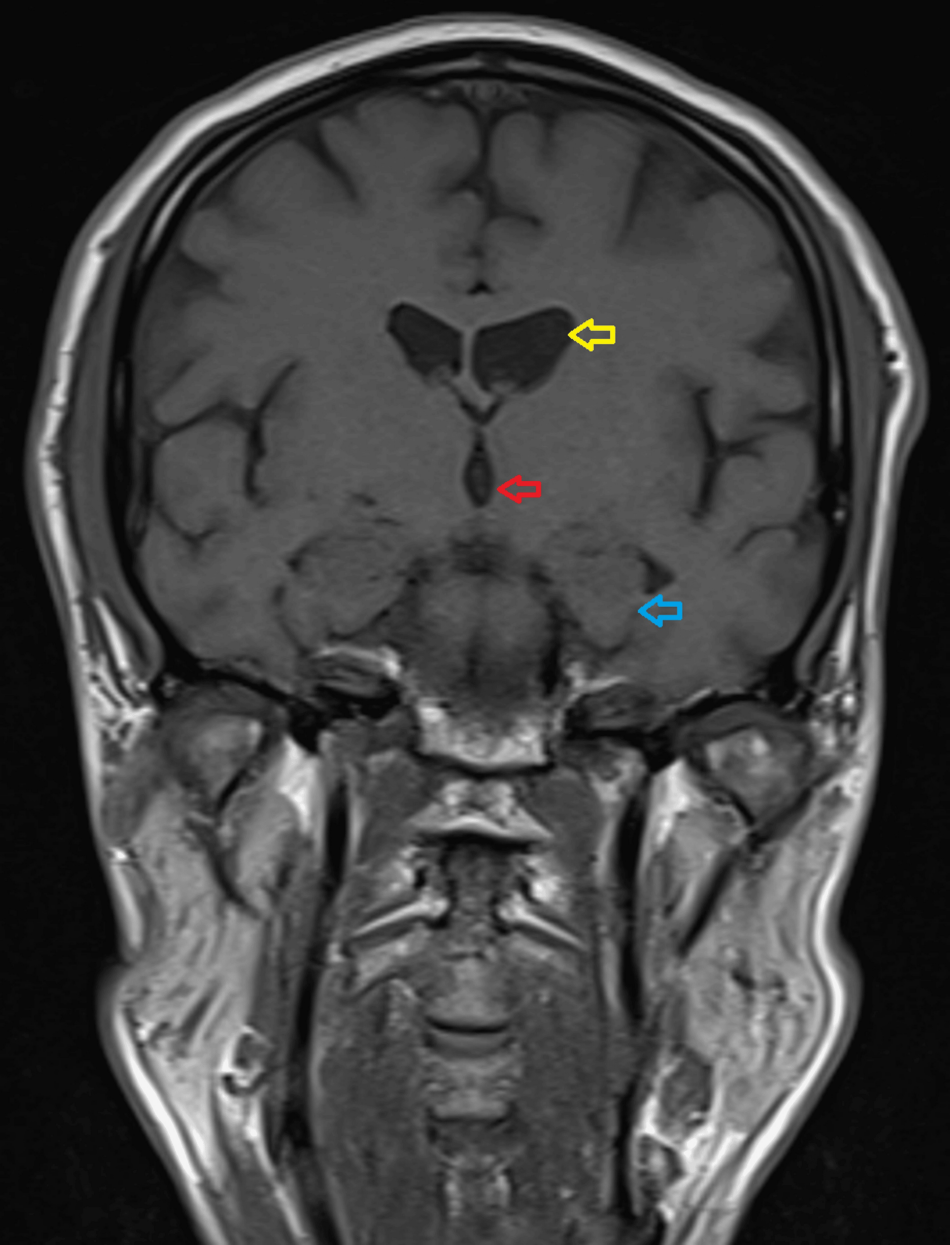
Coronal brain MRI showing the mild enlargement of the lateral ventricles (yellow arrow), minimal enlargement of the third ventricle (red arrow), and normal hippocampus volume (blue arrow)
